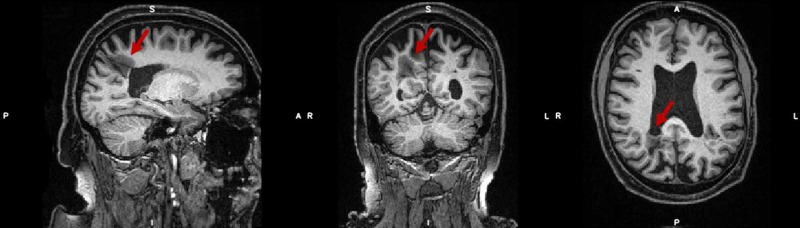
MRI scan (T1-MPRAGE) performed at 3T during research visit The hypo-intense area in the right hemisphere (indicated by the red arrows) measures approximately 13 x 20 x 26 mm (x, y, z). MRI: magnetic resonance imaging; T1‐MPRAGE: T1‐weighted magnetization‐prepared rapid gradient echo.
